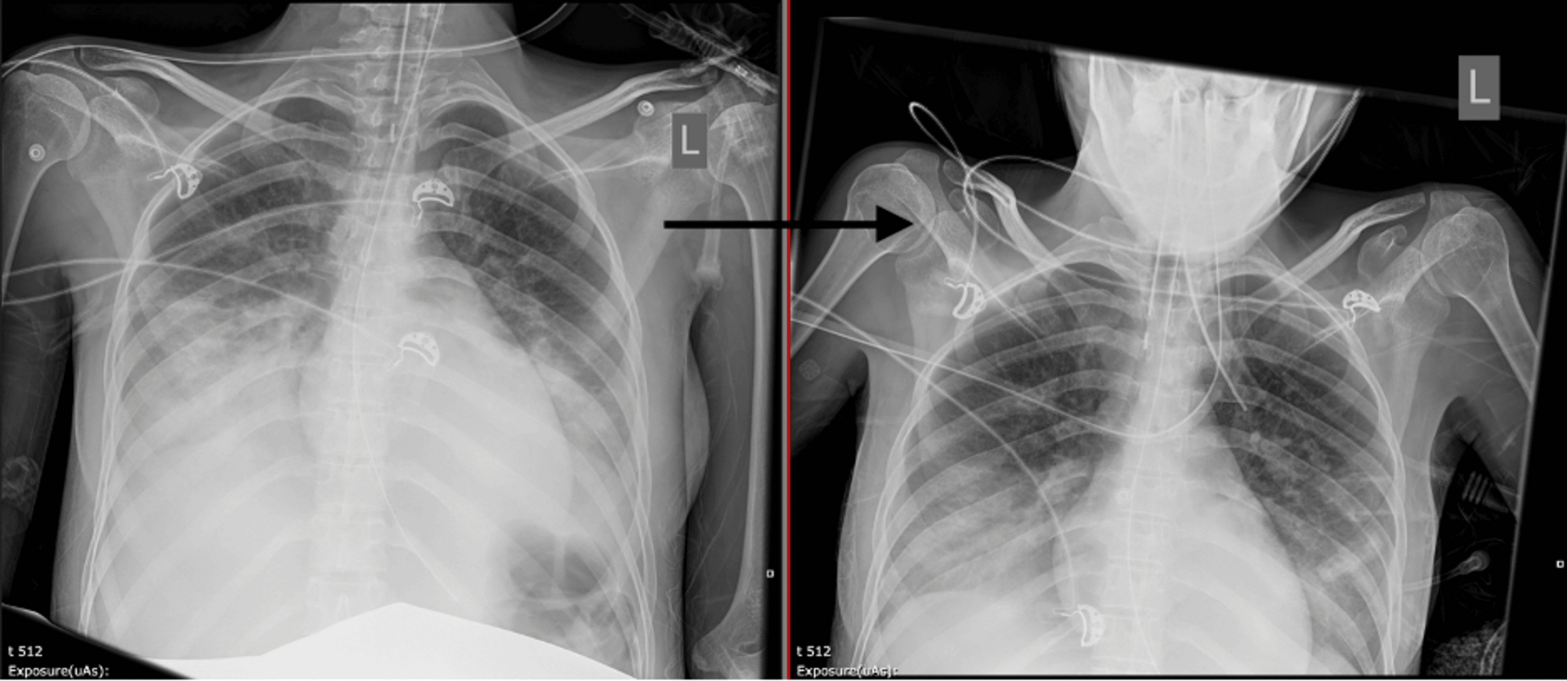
Chest X-ray on the right shows improvement in pulmonary edema and pleural effusion after diuresis.
